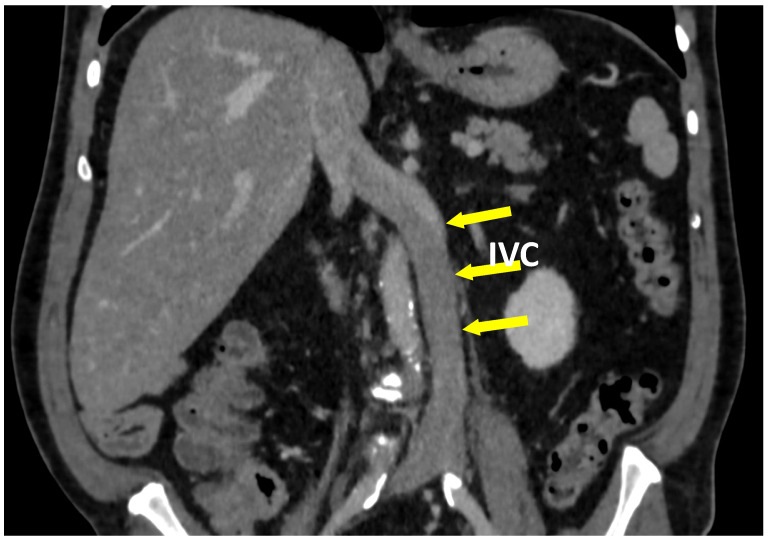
The coronal plane of a CT-V scan in a patient with left-sided inferior vena cava (IVC).
